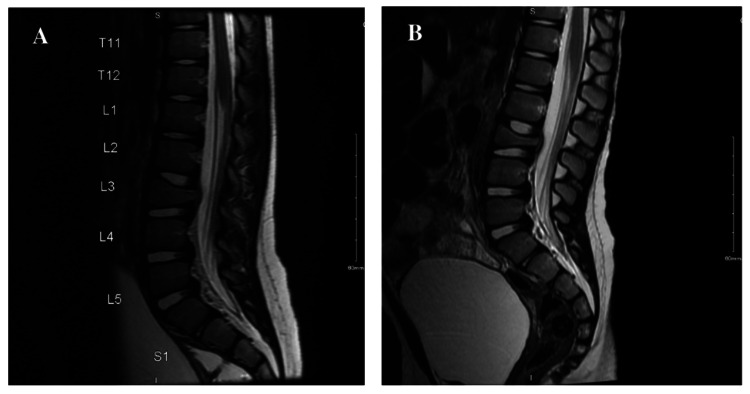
Initial and follow-up L-spine MRI A) Sagittal T2 sequence from the initial L-spine MRI demonstrating altered T1 marrow signal at the L3 vertebral body and posterior elements, significant height loss of the posterior aspect of the L3 vertebral body, and extraosseous involvement at the anterior epidural region with at least minimal/mild thecal sac encroachment. B) Sagittal T2 sequence from follow-up L-spine MRI eight days later demonstrating mild progression of the L3 vertebral body compression deformity.
